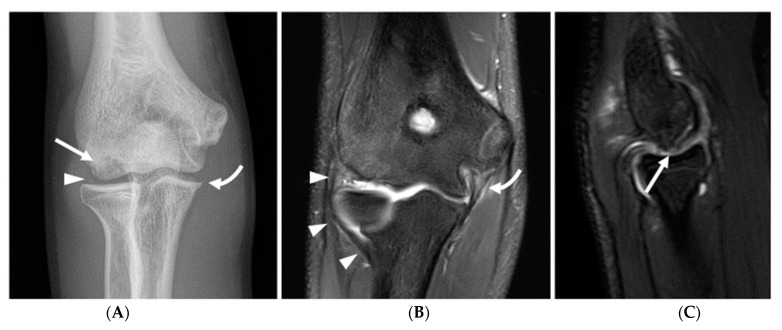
A 16-year-old male right-hand-dominant baseball pitcher presenting with chronic right lateral elbow pain and acute medial pain after pitching. (A) AP radiograph demonstrating irregular lucency in the capitellum (arrow), compatible with osteochondritis dissecans (OCD), and a punctate joint body is seen in the radiocapitellar joint space (arrowhead). A small ulnar traction spur is visible along the sublime tubercle (curved arrow). (B) Coronal fat-saturated proton-density-weighted image demonstrates thickening and of the ulnar collateral ligament (curved arrow), with intrinsic high T2 signal and periligamentous edema, compatible with grade 2 injury. Of note, the lateral ulnar collateral ligament is intact and of normal morphology (arrowheads). (C) Sagittal fat-saturated T2 Cube image demonstrates an osteochondral lesion along the anterior capitellum with disruption of the articular cartilage and subchondral bone plate (arrow), and high T2 signal deep to the subchondral bone plate, indicating an unstable lesion.
